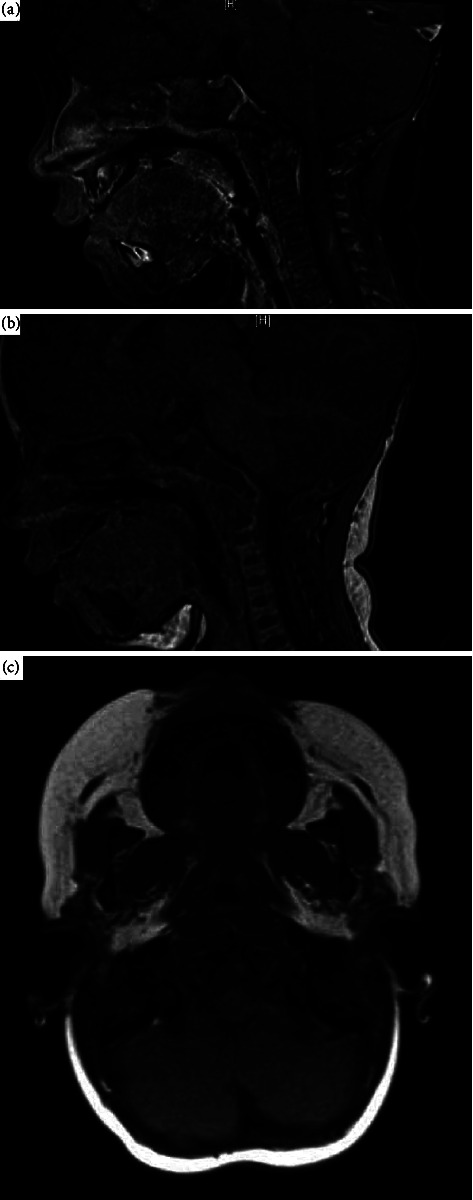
Magnetic resonance imaging (T1 with contrast, (a) 3D MPRAGE, (b) T1 without contrast), and (c) of the head demonstrating a polypoid dorsal midline lingual mass.
